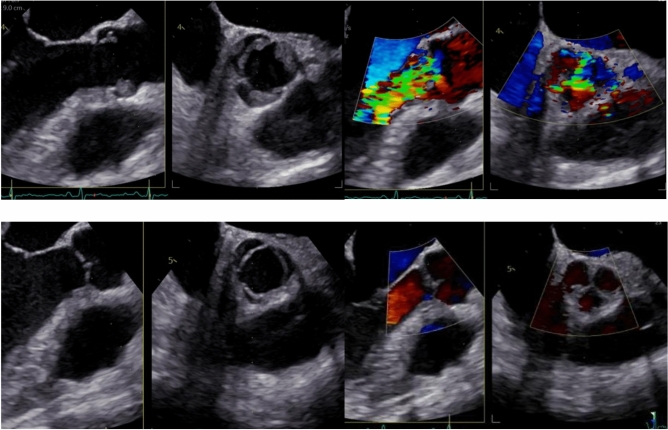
TEE changes in relation to anticoagulation in one NBTE patient. TEE revealing NBTE affecting the aortic valve, accompanied by severe aortic regurgitation in a patient receiving DOAC. Anticoagulation was then transitioned to LMWH, after which serial TEE examinations demonstrated complete regression of the aortic vegetations, including resolution of the regurgitation. Abbreviations: NBTE, nonbacterial thrombotic endocarditis; TEE, transesophageal echocardiography; LMWH, low-molecular-weight heparin.
